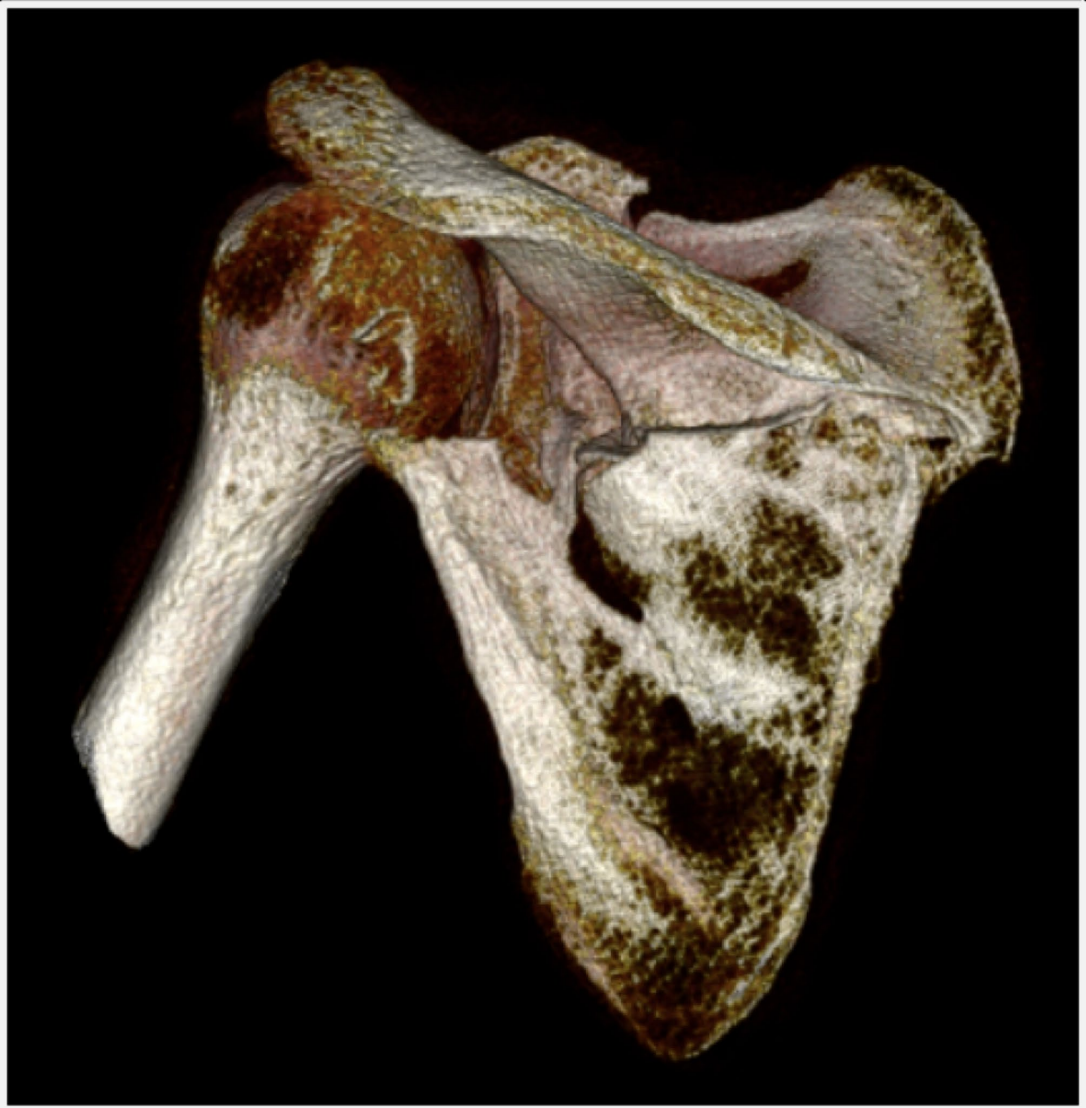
preoperative CT scan of the left scapula and humerus showing impingement between the lateral most prominent spur of the scapular body and the humerus
